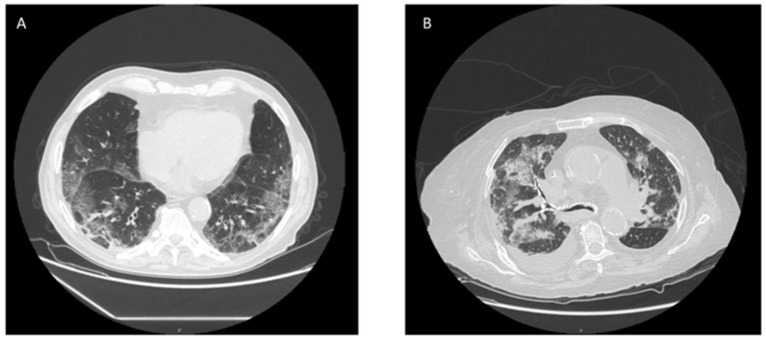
(A) HRCT (patient 1): Bilateral parenchymal thickening with predominantly ground-glass density. (B) HRCT (patient 2): Multiple areas of parenchymal lung thickening such as ground glass, bilaterally and ubiquitously distributed.
